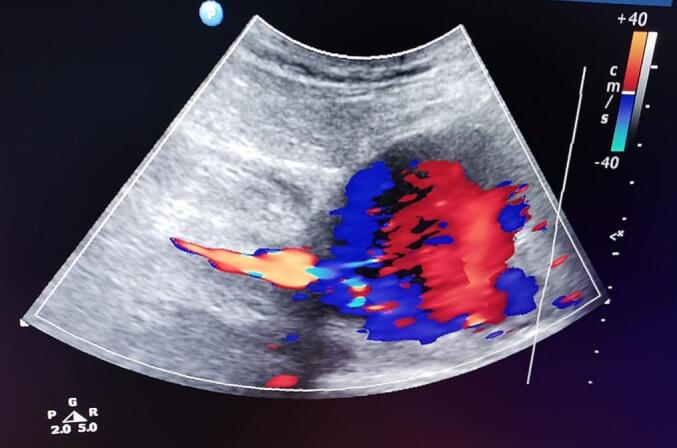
A Color Doppler Ultrasound showed about 80 × 75 × 70 mm pseudoaneurysm at the mid-level of the right SFA with a wide neck leading to compression of the middle segment of the SFA.
